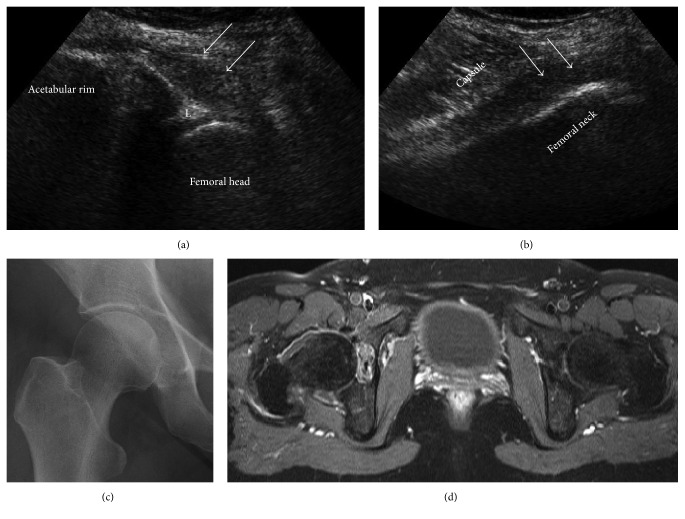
Diagnostic path for patient #4, a 55-year-old woman with a 3-day history of acute pain in the right hip. Ultrasound scans revealed an intra-articular effusion (white arrows; L = labrum) with distension of the capsule (a, b) whereas the radiograph was unremarkable. T2 weighted MRI scans (d) confirmed effusion in the right hip and ruled out extra-articular abscess formation and advanced osteochondral involvement.
